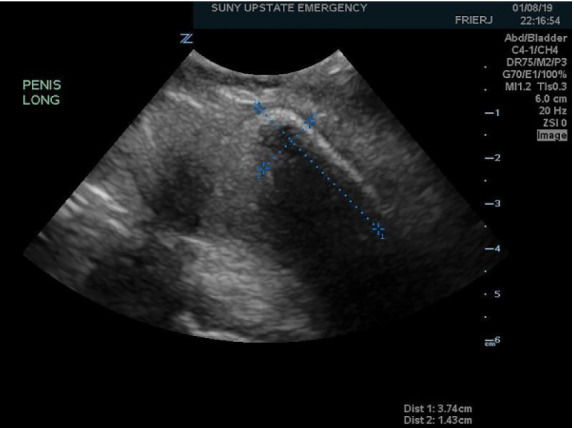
Urethral foreign body measuring 3.74 × 1.43 centimeters, as measured in long axis on point-of-care ultrasound.
